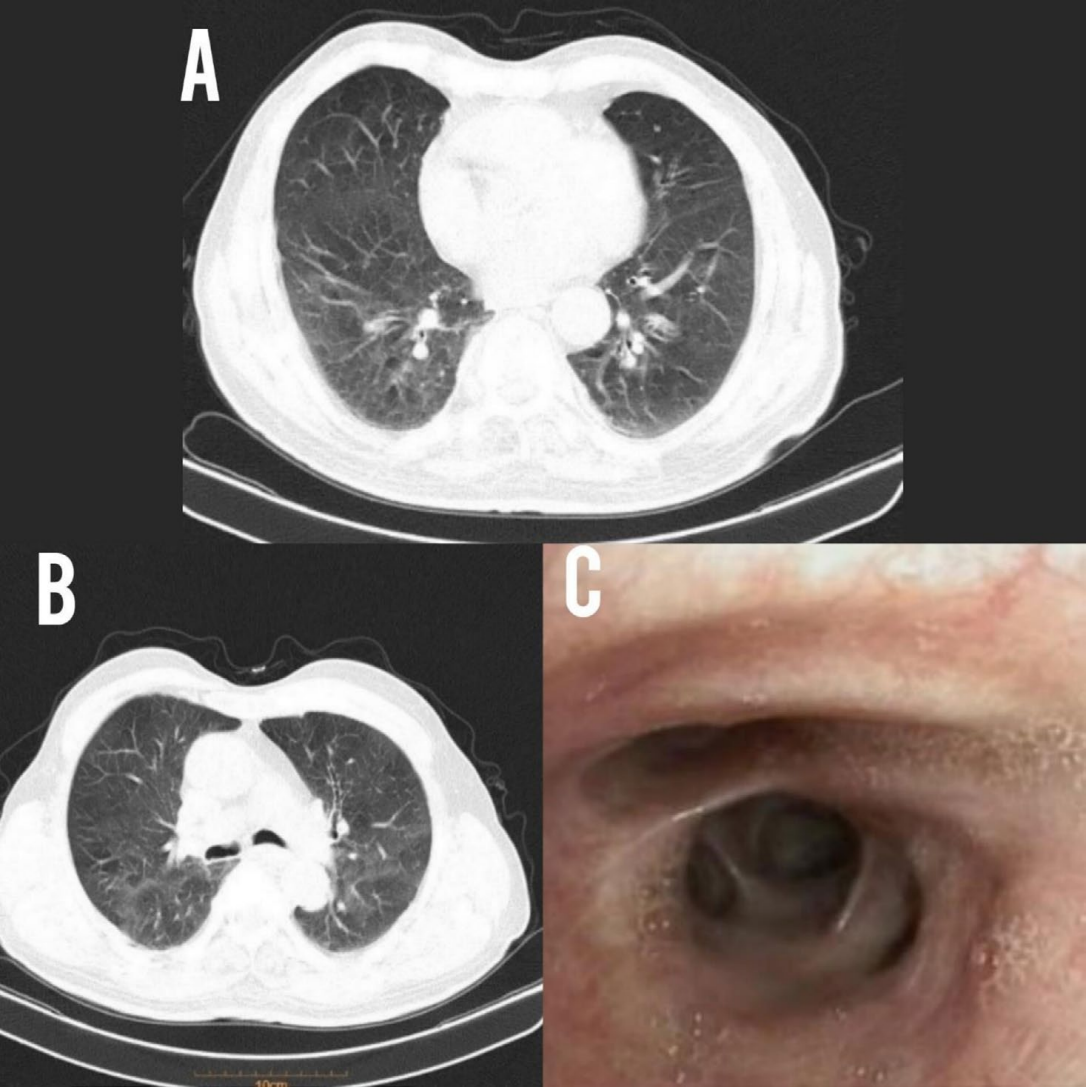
(A and B) Sections of spiral lung CT scan reveal mosaicism and bronchial wall thickening; (C) bronchoscopic view of intermediate bronchus reveals bronchial erythema.
